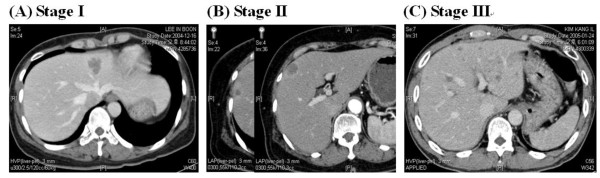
Pettavel and Morgenthaler's Staging for colorectal hepatic metastases. (A) CT imaging for stage I, (B) CT imaging for stage II, and (C) MRI imaging for stage III disease.
